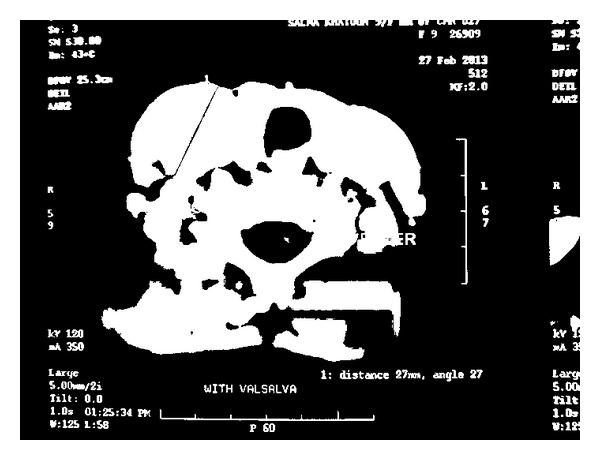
A contrast enhanced CT scan showing ectasia of the right internal jugular vein.
